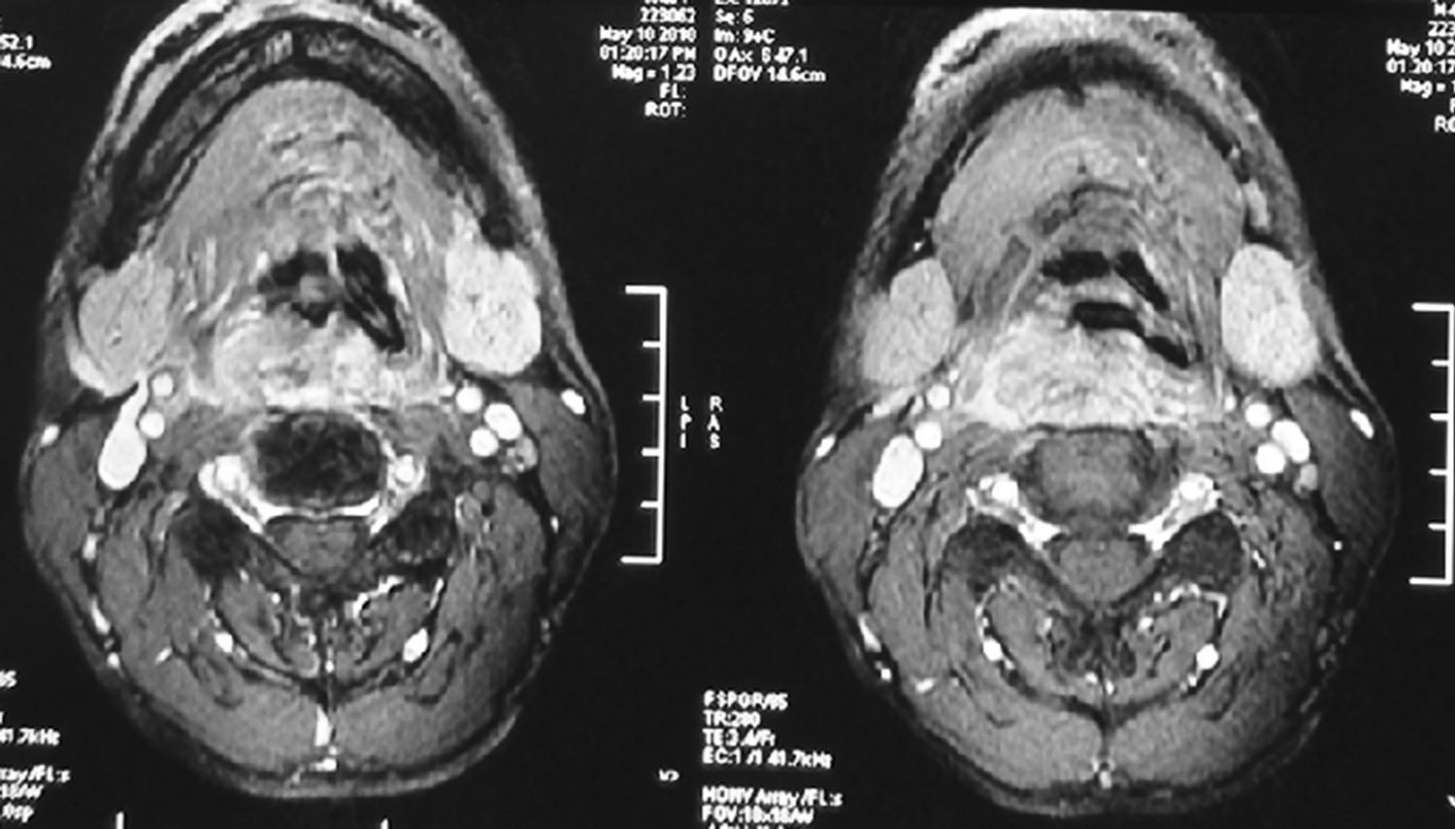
Preoperative MRI examination in a patient with a right piriform sinus lesion involving the cervical esophagus and oropharynx
